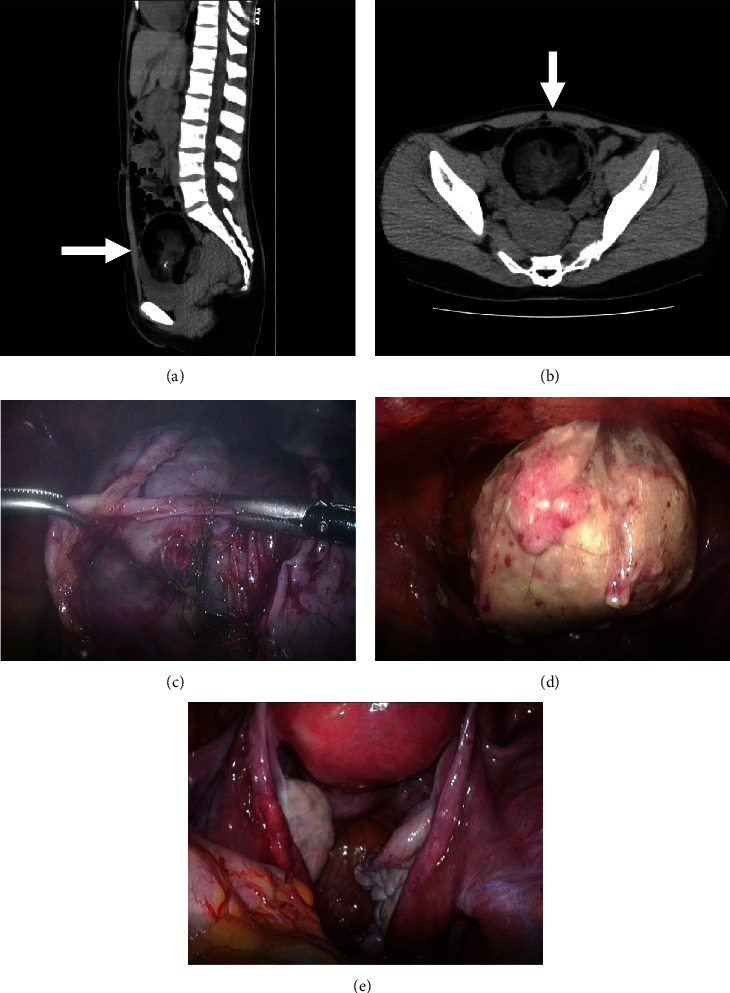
Pelvic computed tomography showing a dermoid cyst. No malignant findings (a, b, white arrow) are noted. Intraoperatively (c), the cyst was removed from the body using a bag (d), and the pelvic cavity was cleaned (e).
